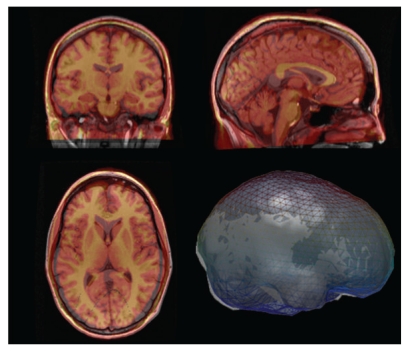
The overlay of the warped template MRI (red/yellow) with the actual MRI (grey) shows a reasonable fit of the scalp surface and neuro-anatomy. A mesh overlay of the warped template brain (blue) and the actual brain (grey) is also shown.
